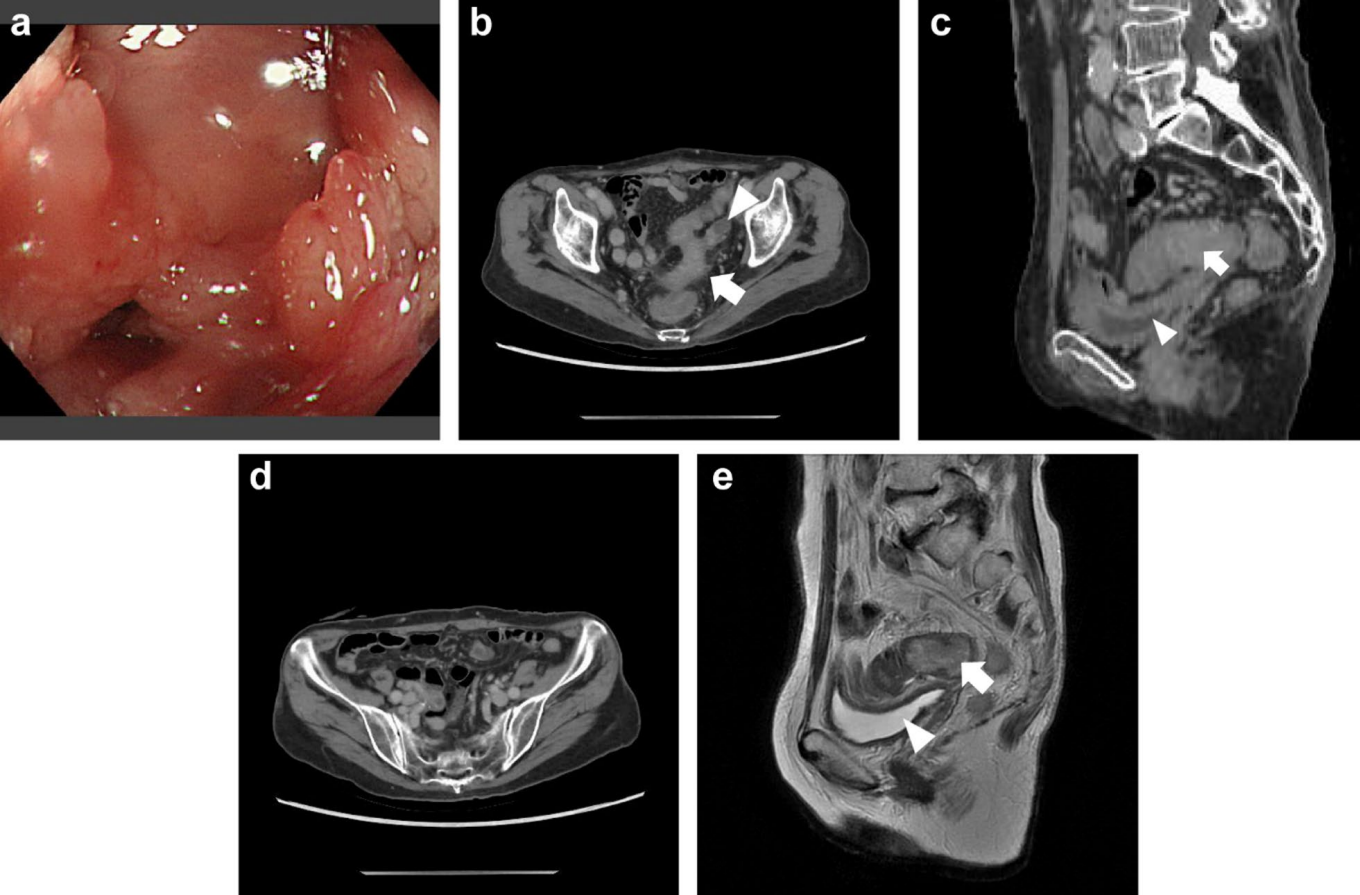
Examinations after preoperative chemotherapy. The tumor shrank with flattening on colonoscopy (a). On CT, the tumor also shrank remarkably (arrow), although invasion of the left ovary (arrowhead) was still possible (b). The fat layer between the tumor (arrow) and the bladder (arrowhead) was observed (c). Enlarged regional lymph nodes were disappeared (same slice level as Fig. 2d (d). Magnetic resonance imaging also revealed that invasion of the bladder was negative: the fat layer between the tumor (arrow) and the bladder (arrowhead) was preserved (e)
